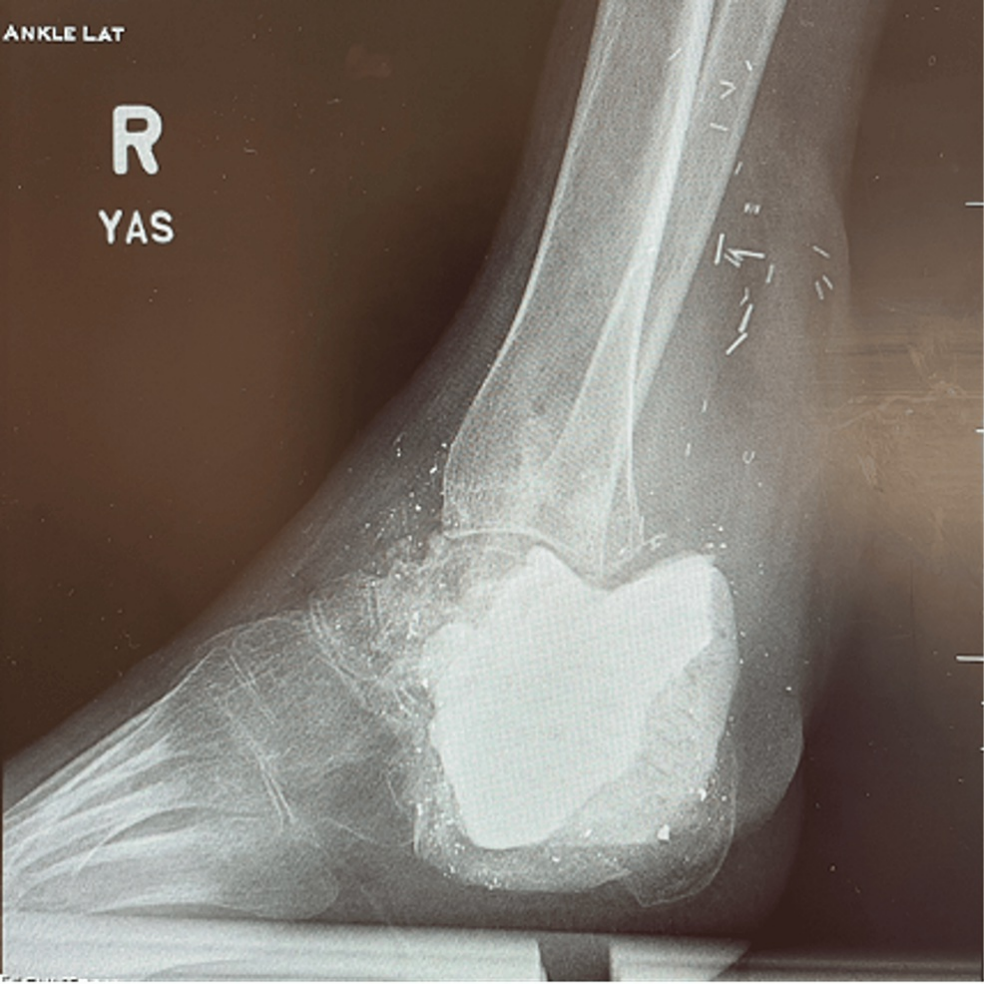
Radiograph after completion of stage 1 with antibiotic cement filler.
